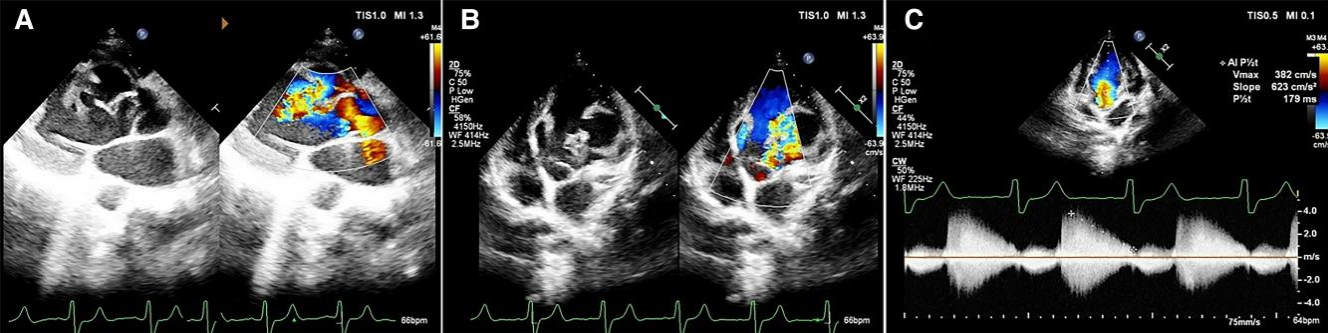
(*A–C*) Transthoracic echocardiogram. (*A*) Parasternal long-axis view with colour Doppler showing ruptured sinus of Valsalva from the right coronary sinus into the interventricular septum and opening into the left ventricle. (*B*) Apical five-chamber view with colour Doppler showing two separate sinus of Valsalva aneurysms from the right and left coronary sinuses rupturing into the left ventricle. (*C*) Continuous wave Doppler at the site of rupture of aneurysm showing diastolic flow into the left ventricle with pressure half time of 179 ms suggestive of severe aortic regurgitation.
